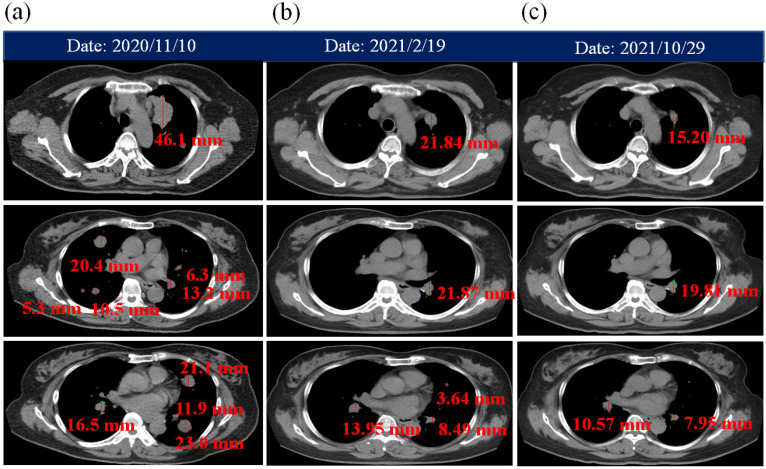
(a) Multiple pulmonary metastases of bilateral lung by chest CT. The CT scans of the patient whom received (b) 2 and (c) 10 months of combined therapy.
